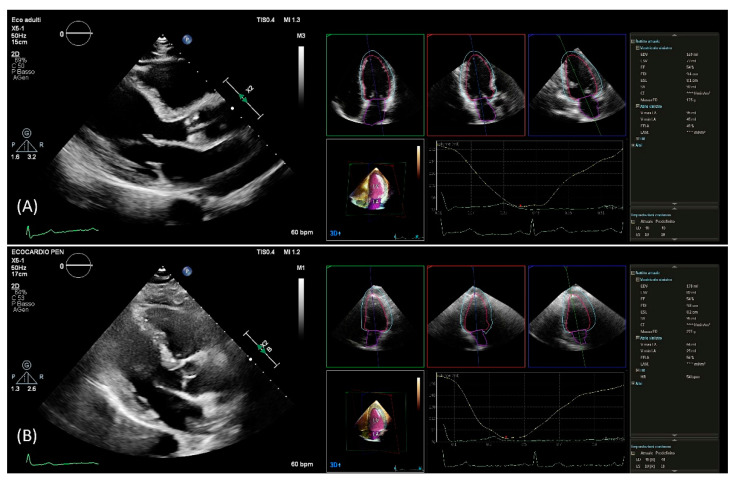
PLAX view used for linear 2D measurements and 3D measurements obtained from automated DHM software in a patient with good agreement (A) and in a patient with poor agreement (B) between 2D and 3D mass values. DHM, Dynamic Heart Model; PLAX, parasternal long axis; 2D, two-dimension; 3D, three-dimension.
